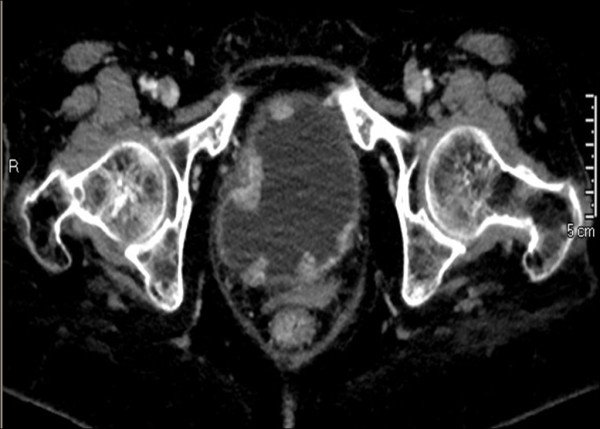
Abdominal computed tomography. Several solid lesions protruding into the bladder lumen are seen.
